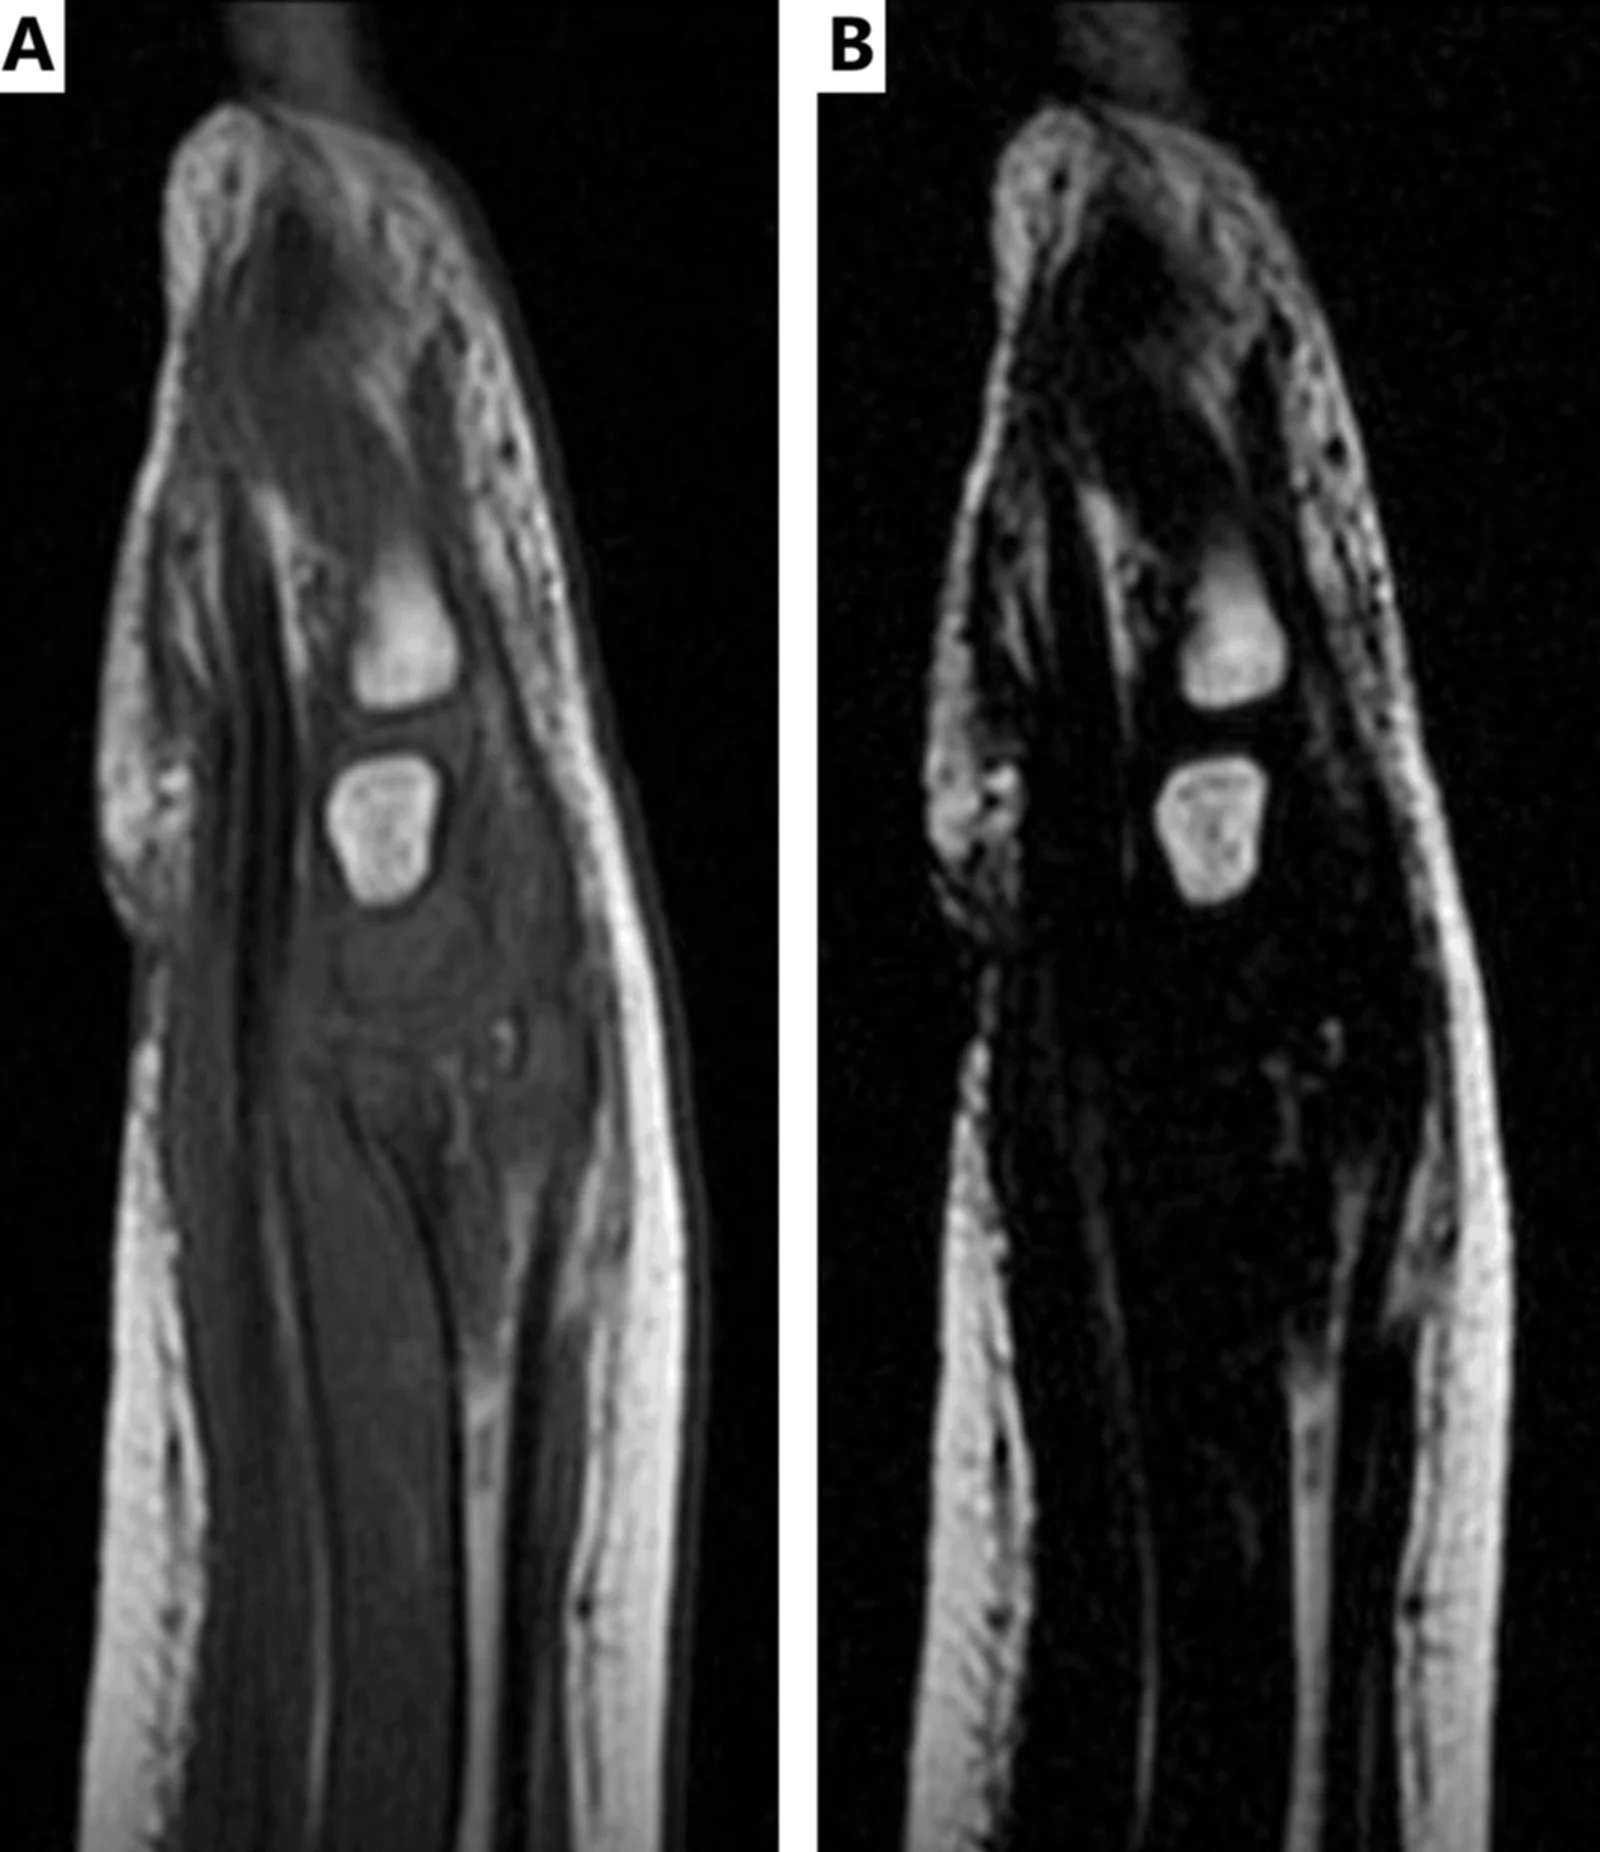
Magnetic resonance imaging of the left forearm at admission The images are displayed with the distal end (fingertips) at the top to match the orientation of the plain radiographs, as shown in Figure 2. (A) T1WI demonstrates a hypointense signal in the distal left radius. (B) Fat-sat T2WI of the same level shows heterogeneous high signal intensity, consistent with osteomyelitis. Edematous changes are also observed in the volar soft tissues of the wrist T1WI: T1-weighted image; Fat-sat T2WI: fat-suppressed T2-weighted image
